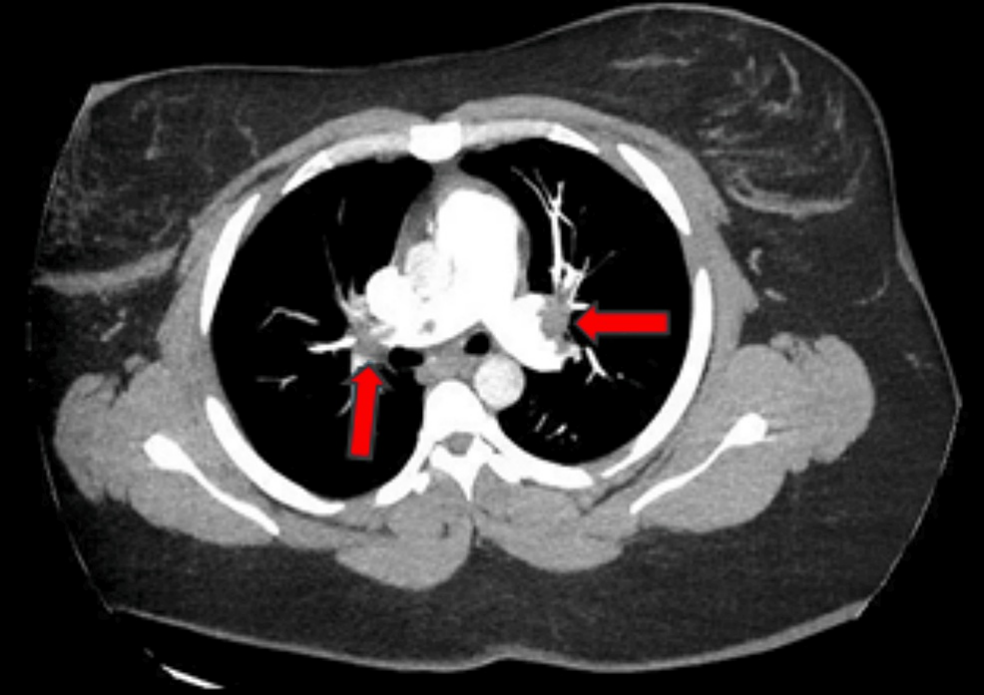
Repeat CT angiogram showing decreased saddle pulmonary embolism but significant intraluminal defects in multiple lobes (red arrows).
